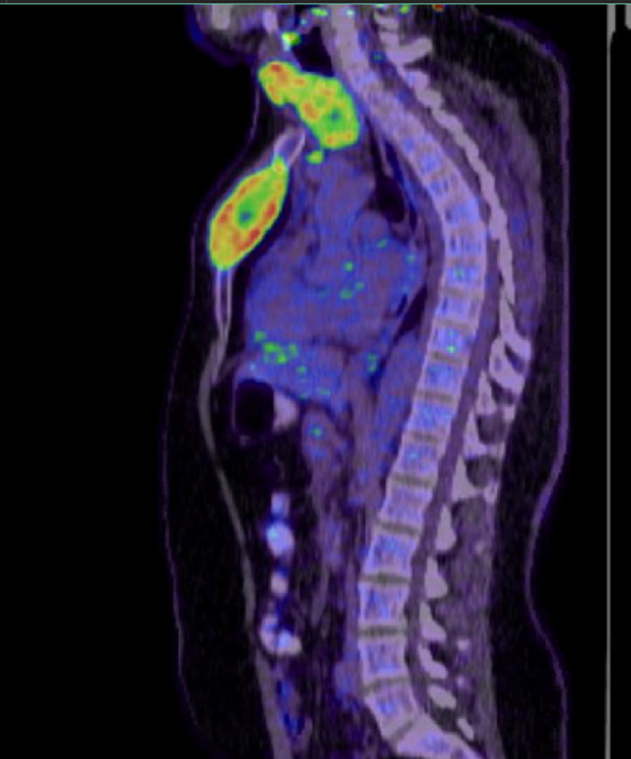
PET-CT scan: showing intensely hypermetabolic expansile lytic lesion in the sternum.
